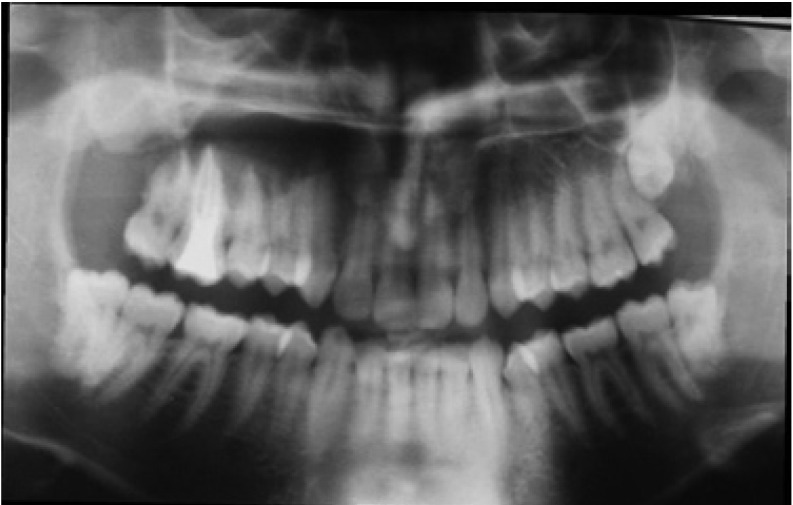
Panoramic radiograph of a KCOT occupying the right maxillary sinus, between the right second incisor and the second molar teeth. The border of the lesion is not readily apparent
